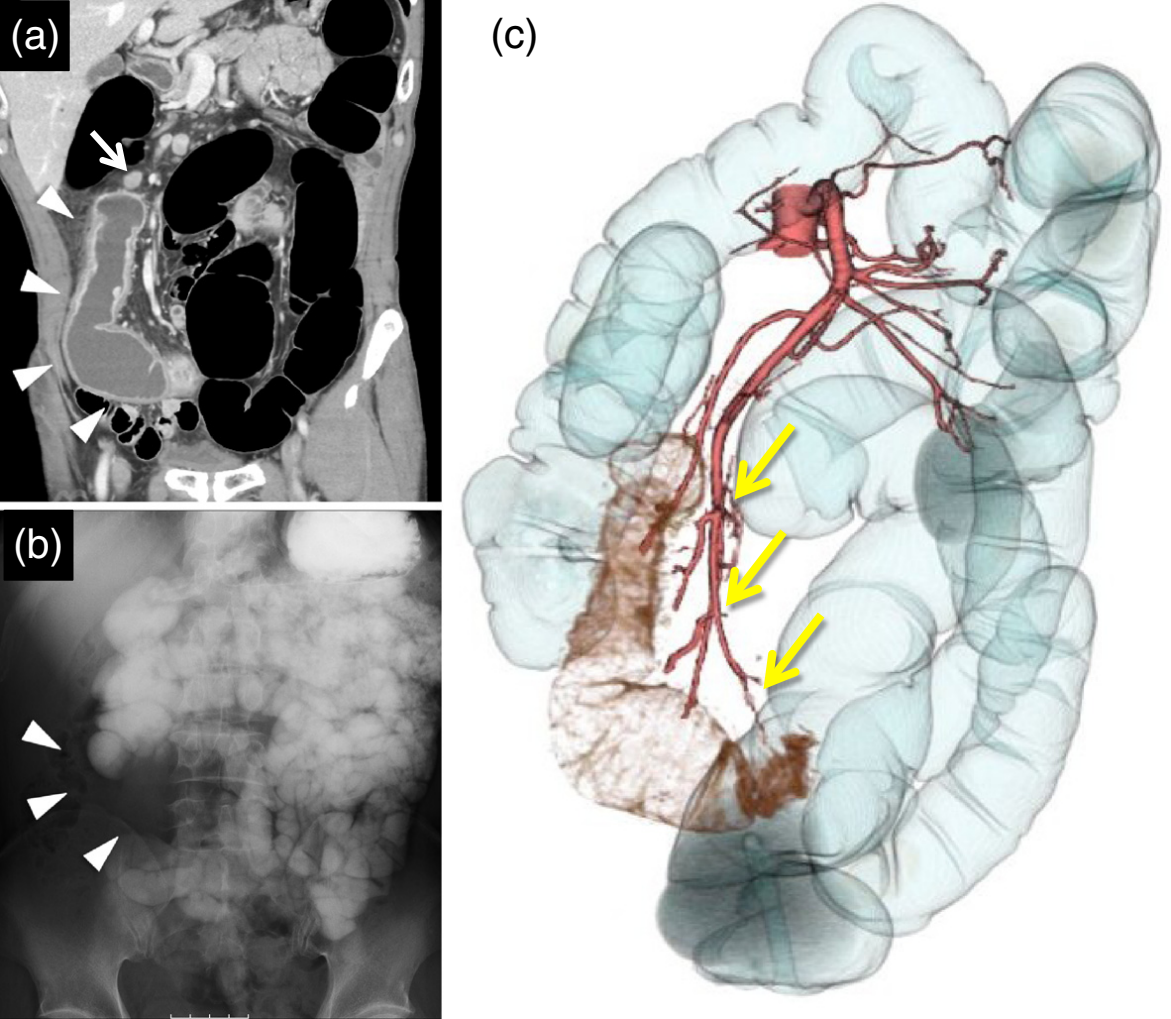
Coronal reconstruction image, small bowel follow-through exam, and 3d reconstruction image. (a) A coronal reconstruction image of a CT scan showed a tortuous tubular cyst that was 15 cm in diameter (arrowhead), and swelling of the lymph nodes was detected around the cyst (arrow). (b) A small bowel follow-through exam showed that the cyst did not come out, but that it excluded normal intestine (arrowhead). (c) A 3D reconstruction image showed that the feeding arteries were separated from the upper mesentric artery (arrow).
